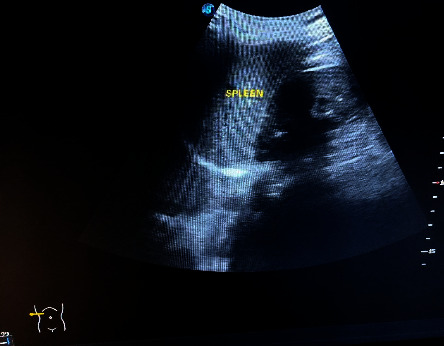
A right-sided spleen is shown in an ultrasonography image of the right hypochondrium.
